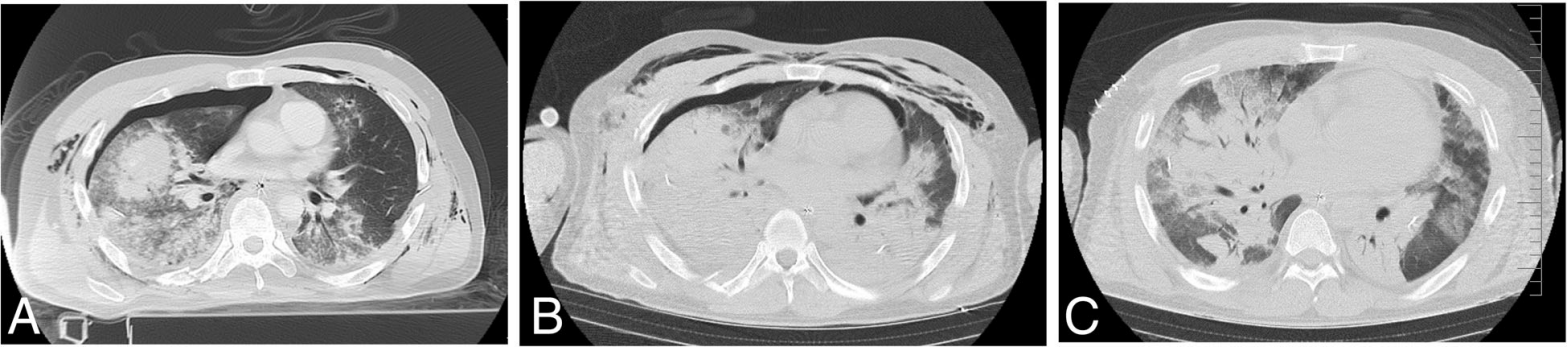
Chest CT images during the course of treatment: a initial; b postthoracotomy; c day 8 after heparin-free ECMO. Bleeding from bilateral lung contusions decreased before and after heparin-free ECMO
